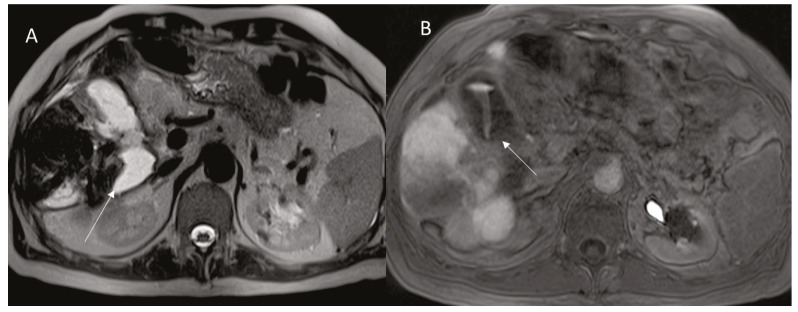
Man 74 year at 1-month follow-up after RFA of HCC on VI seg. MRI ((A): HASTE T2-W sequences in axial, in (B): EOB-phase of contrast study in axial plane). The arrow shows bile leak.
